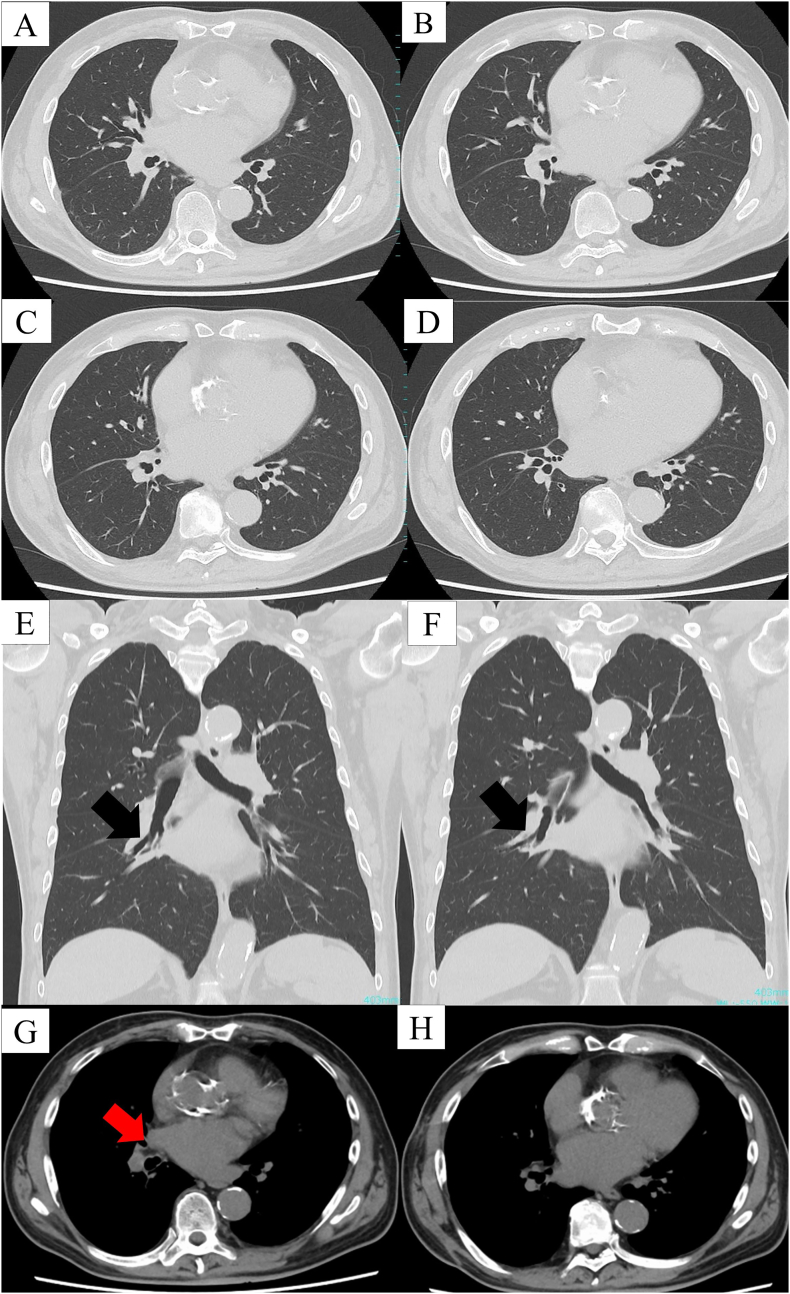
Six-months postoperative computed tomographic (CT) images are shown. (A–H) Six-months postoperative CT demonstrating no evidence of stenosis at the site of the sutured bronchus. The right lung is fully expanded. A black arrow shows the right anterior basal segmental bronchus. A red arrow is indicative of the pericardial flat flap. (For interpretation of the references to colour in this figure legend, the reader is referred to the web version of this article.) (For interpretation of the references to colour in this figure legend, the reader is referred to the web version of this article.)
